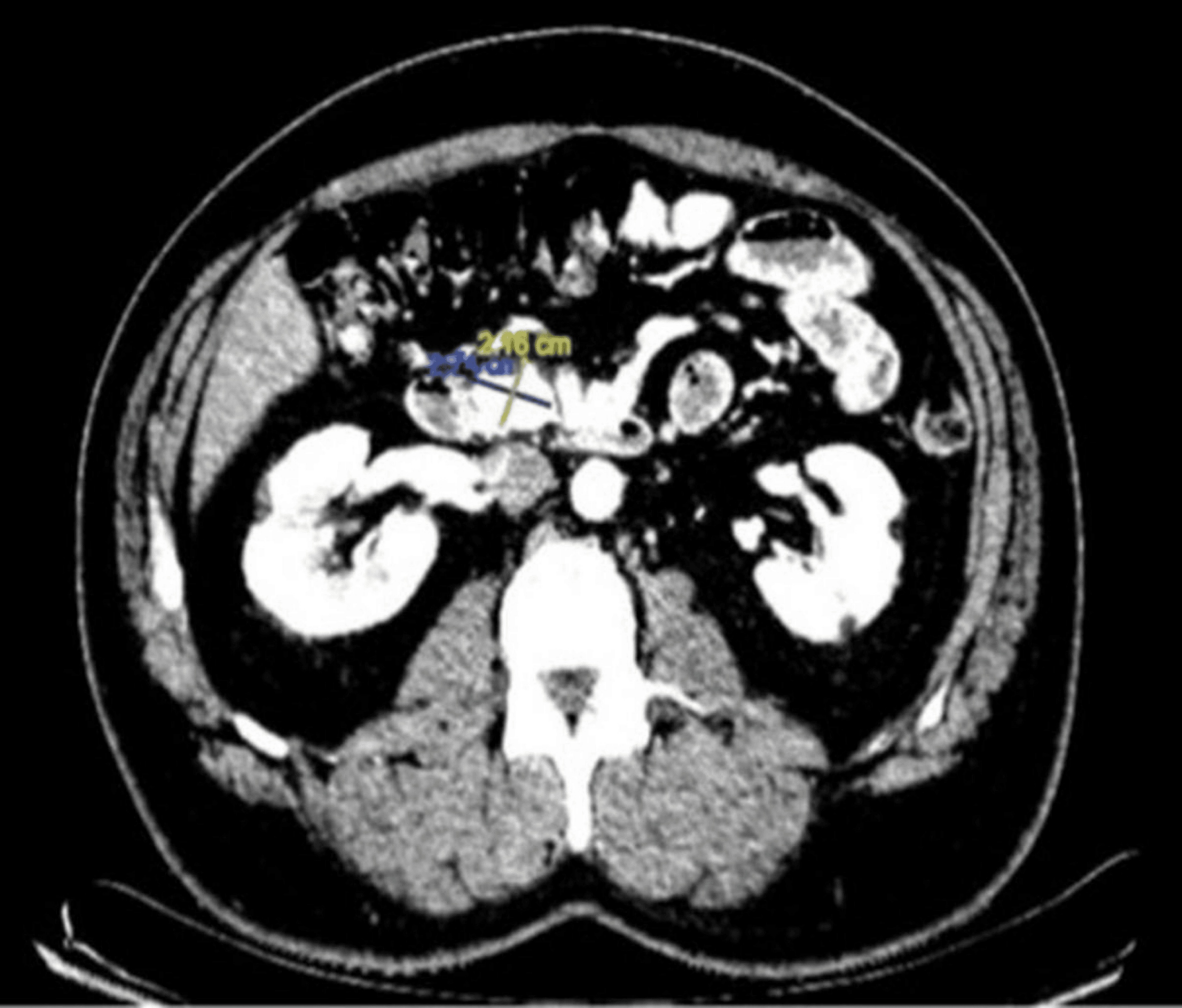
One-year follow-up CT scan CT scan obtained in December 2019, one year post-SBRT, showing tumor regression with reduced size of approximately 2.7 × 2.1 cm. CT: Computed tomography; SBRT: Stereotactic body radiation therapy
